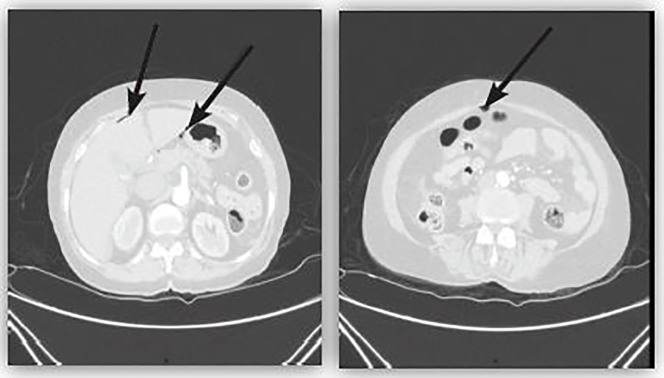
Computed tomography scan showing free air in the abdominal cavity.
